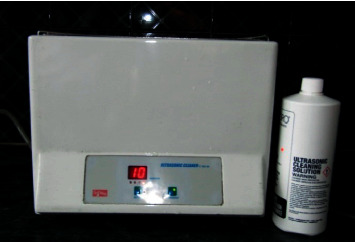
Ultrasonic cleaning of brackets.
